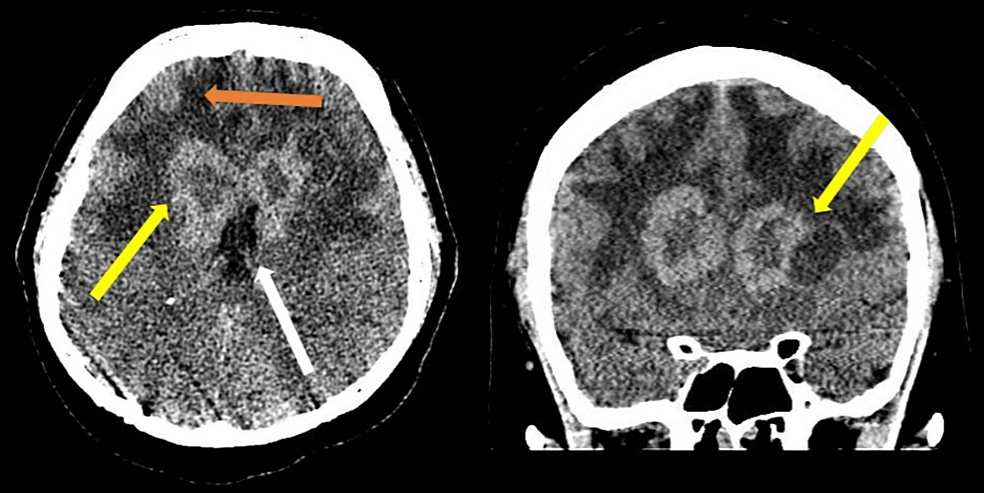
CT images from the base of skull to vertex without IV contrast. There is bilateral frontal lobe periventricular hyperdense lesions with areas of cystic changes (yellow arrows) and extensive surrounding cerebral edema (orange arrow). There is effacement of bilateral lateral ventricles (white arrow).
